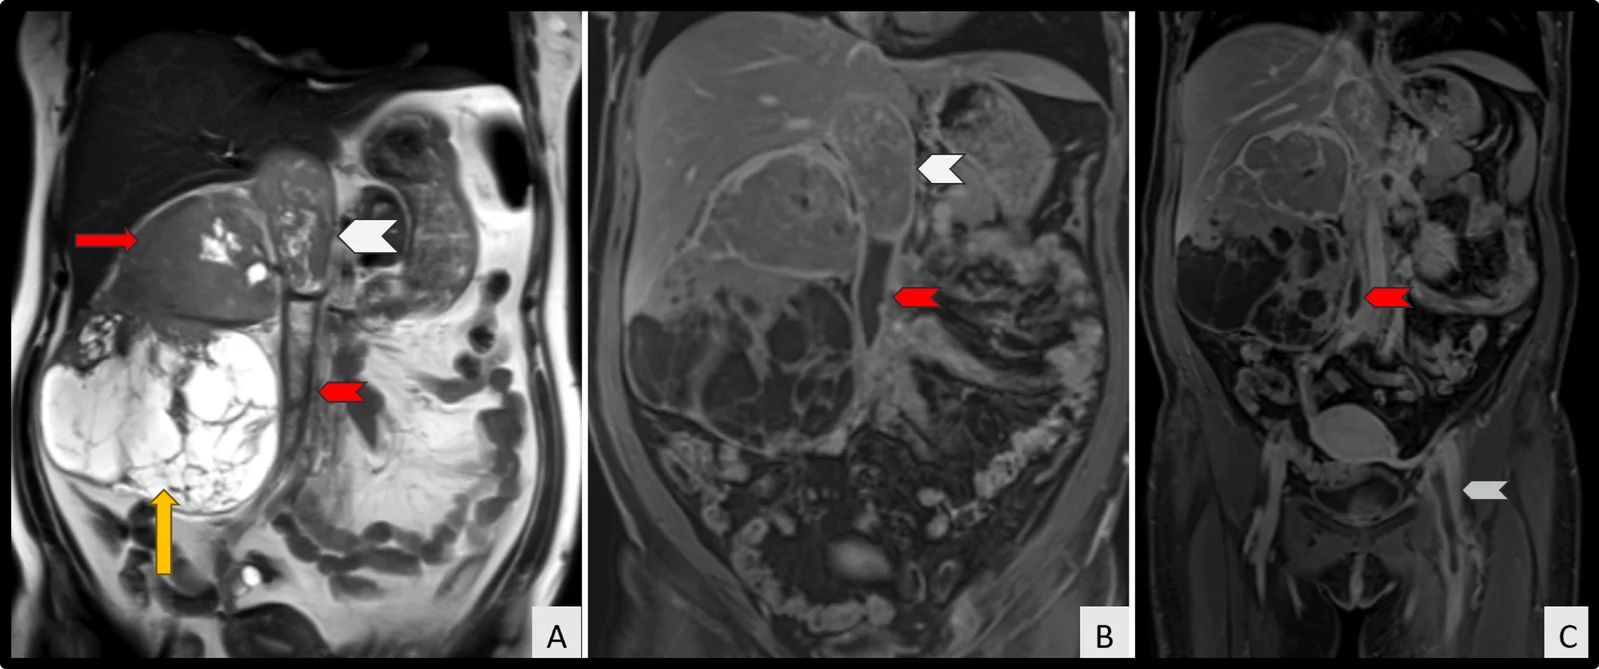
MRI of the primary renal tumor in the right kidney with venous extension A: Coronal reconstructed T2-weighted half-Fourier acquisition single-shot turbo spin echo (HASTE) image demonstrates a large heterogeneous right renal mass (red arrow) occupying the interpolar and upper polar region, with contiguous tumor thrombus extending into the right renal vein and IVC (white arrowhead). Distal to the enhancing tumor thrombus, a bland thrombus (red arrowhead) is identified within the infrahepatic IVC. The yellow arrow points to the large exophytic component of the tumor with a major necrotic component. B: Coronal reconstructed contrast-enhanced T1-weighted image demonstrates heterogeneous enhancement of the primary renal mass and contiguous enhancing tumor thrombus within the IVC (white arrowhead), while the distal infrahepatic bland thrombus remains non-enhancing(red arrowhead), confirming both types of venous thrombosis. C: Coronal reconstructed contrast-enhanced T1-weighted image demonstrates extension of venous thrombosis into the left lower limb deep venous system (green arrowhead), consistent with associated deep venous thrombosis (DVT). IVC: Inferior vena cava
